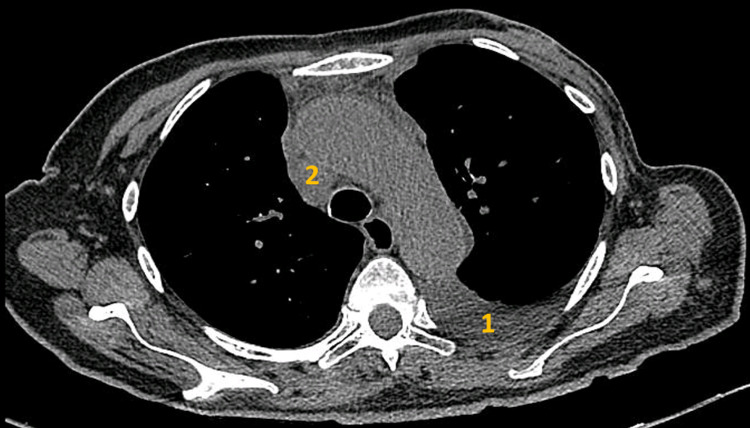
Chest CT showing pleural effusion (1) and mediastinal adenopathy (2).
